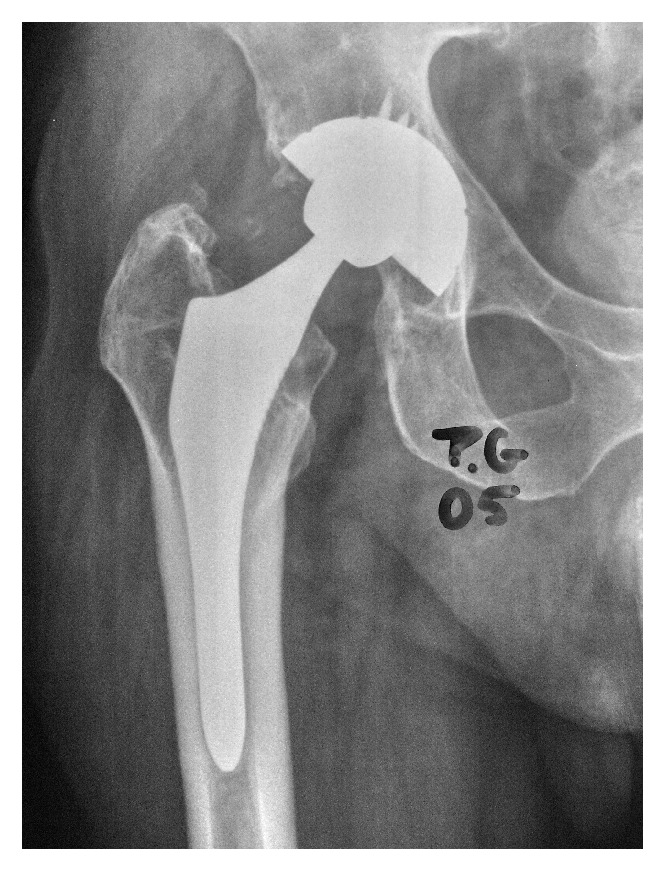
X-ray image of patient with follow-up at 12 y. Osteolysis in acetabulum produced by excessive polyethylene wear.
